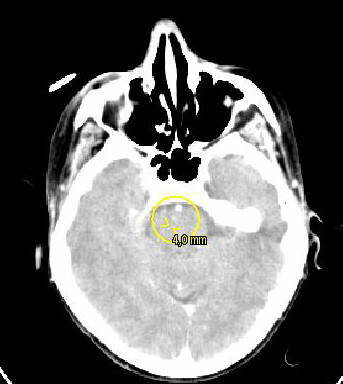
Horizontal section of the head (CT-scan) showing an intracerebral metastasis (diameter 0,4 mm).
